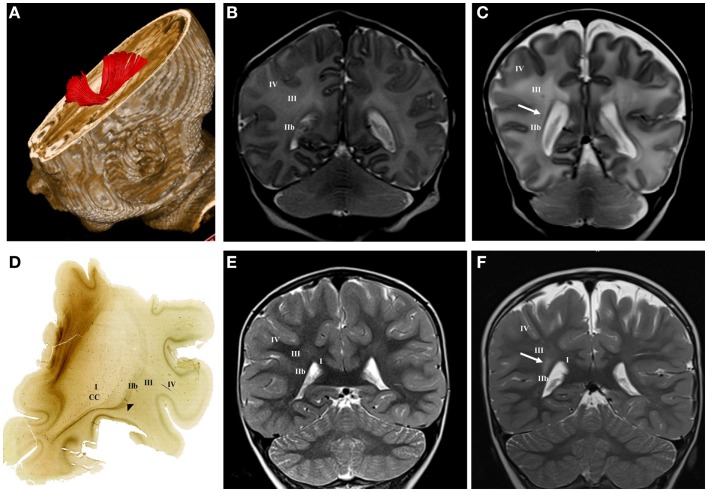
DTI tractography reconstruction of sagittal strata at term age (A). The acetylcholinesterase (AChE) histochemistry shows external sagittal stratum [arrowhead in (D)] at the frontal level at term age. Normal MRI findings of sagittal strata at term age (B) and at the age of 3 years (E) on T2 coronal images. Late preterm child (born at 30 PCW, BW = 1510 g, SNAPII = 16, SNAPPEII = 34, AS = 2/5) with punctate hemorrhagic lesion within sagittal strata at term equivalent age [arrow in (C)] and hyper-intensive lesion of sagittal strata at the age of 3 years [arrow in (F)] on T2 coronal images. Numbers I–IV represent segments of white matter as previously described, CC, corpus callosum.
